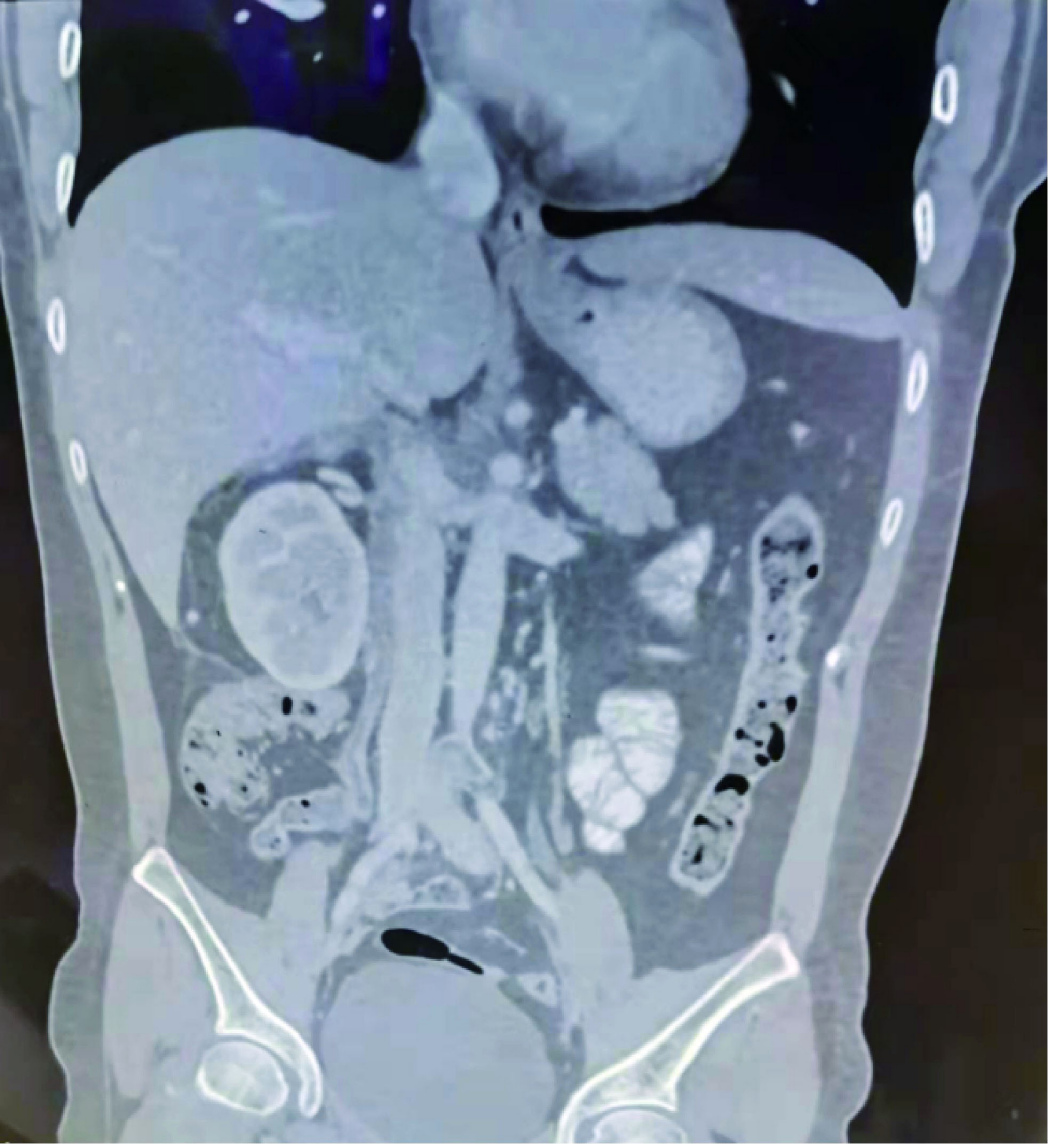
Abdominal CTA 3 months after surgery.
